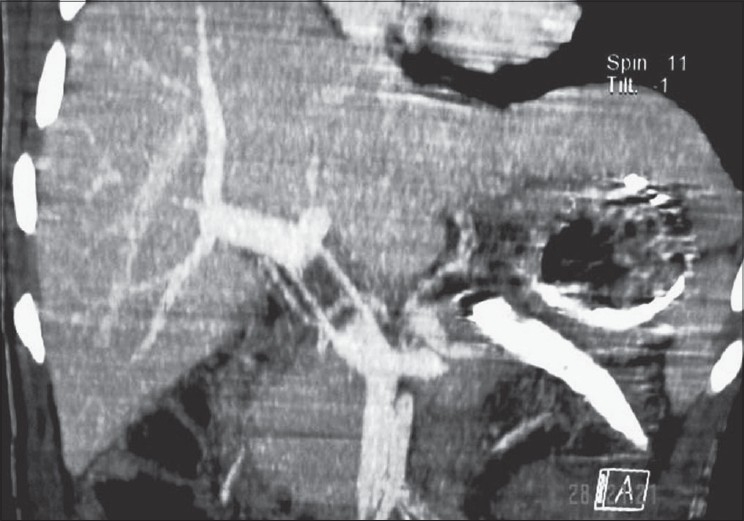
Coronal MPR CT image shows an acute main portal vein thrombus
